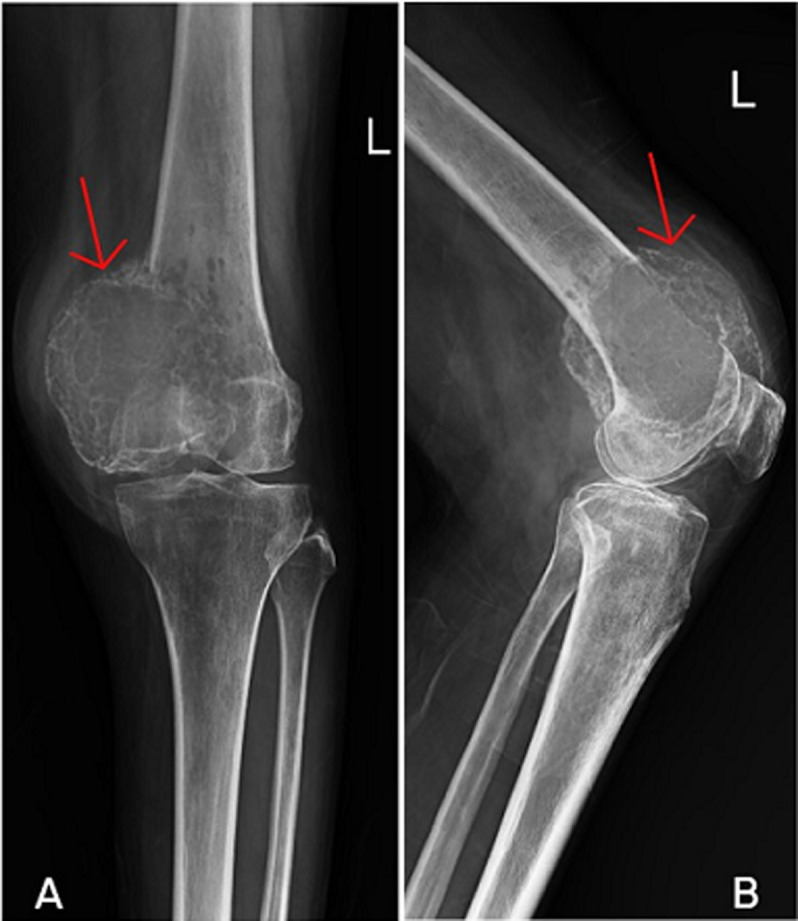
A, B) X-ray showing expansile lytic lesion at the distal femur
